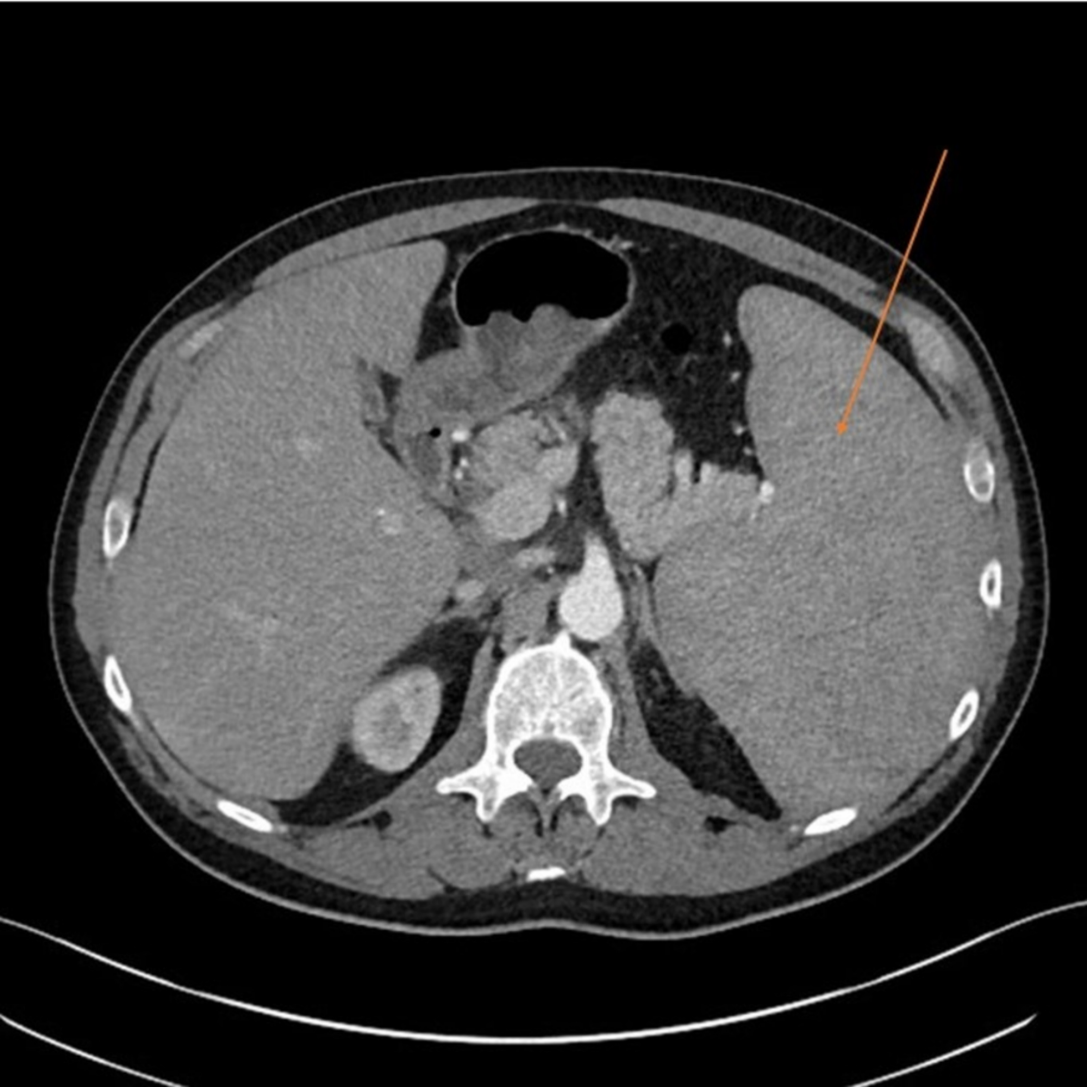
CT abdomen demonstrating severe splenomegaly, craniocaudal length of almost 20 cm.
